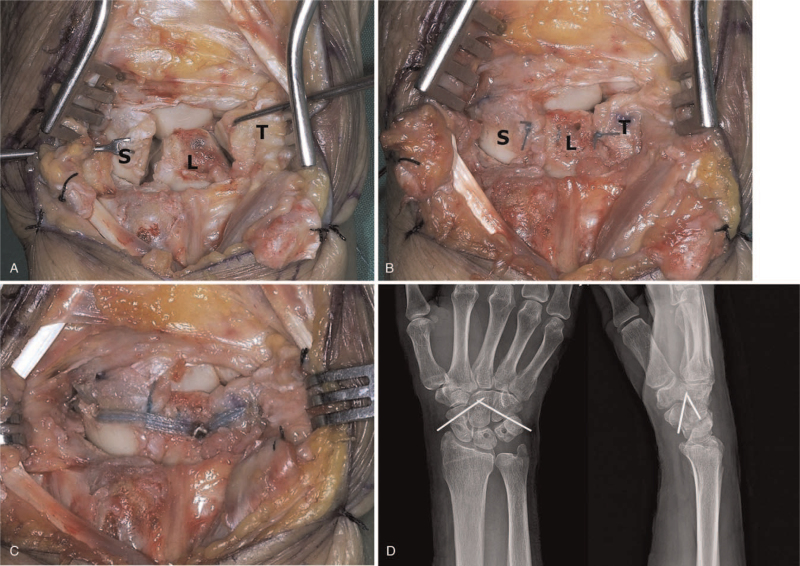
Intraoperative photographs. (A) Complete rupture of the SLIL and LTIL were confirmed. (B) The dorsal SLIL and LTIL were carefully repaired using non-absorbable sutures. However, ligament repair was unreliable and insecure. (C) So we performed IB augmentation using Fibertape to secure the repair. (D) Postoperative radiographs demonstrate a well-reduced carpal alignment. L = lunate, LTIL = lunotriquetral interosseous ligament, S = scaphoid, SLIL = scapholunate interosseous ligament, T = triquetrum.
